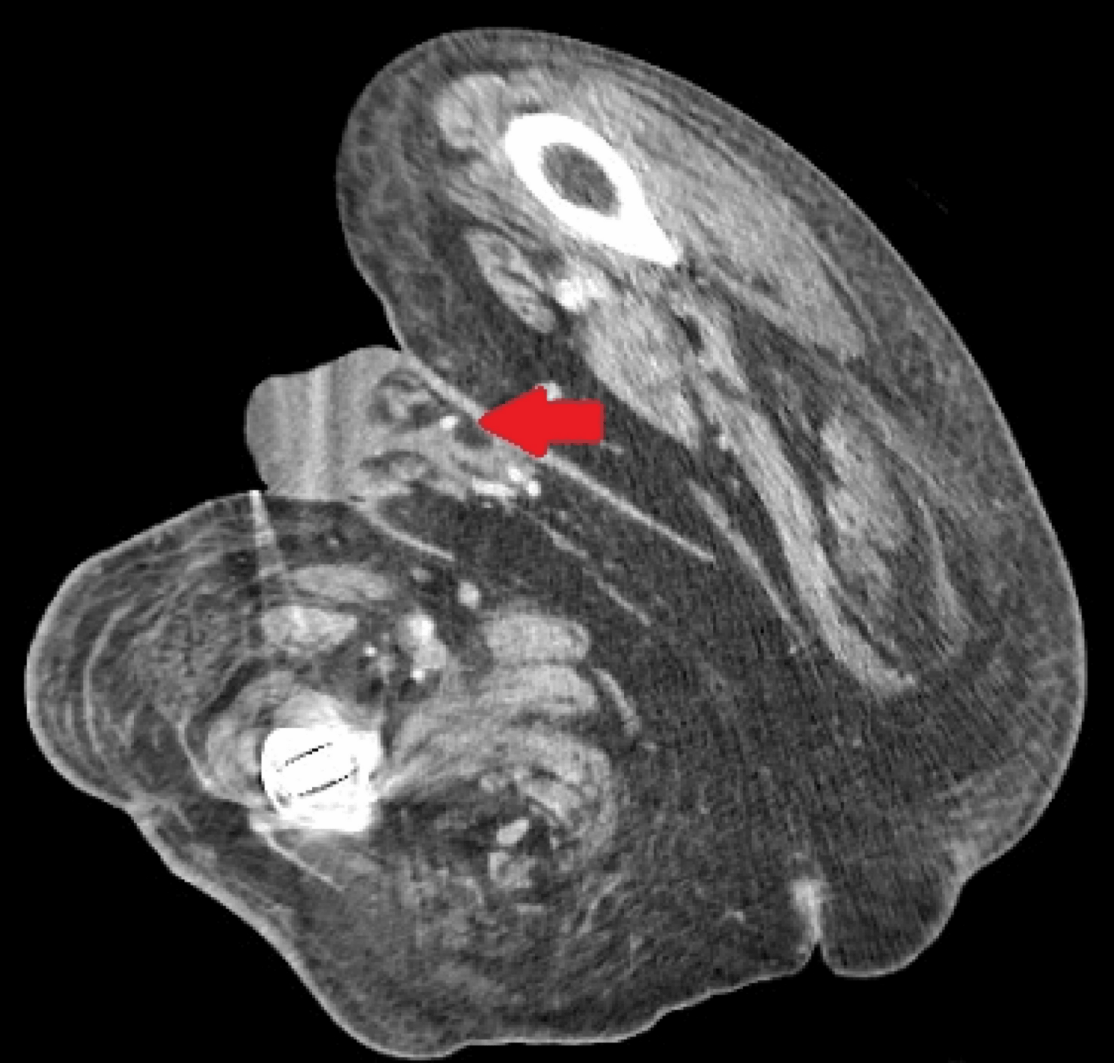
Contrast-enhanced CT (axial) of the pelvis at the level of the penis The red arrow points to the rim-enhancing fluid collection along the inferior aspect of the penile prosthetic cylinders with surrounding inflammatory changes.
